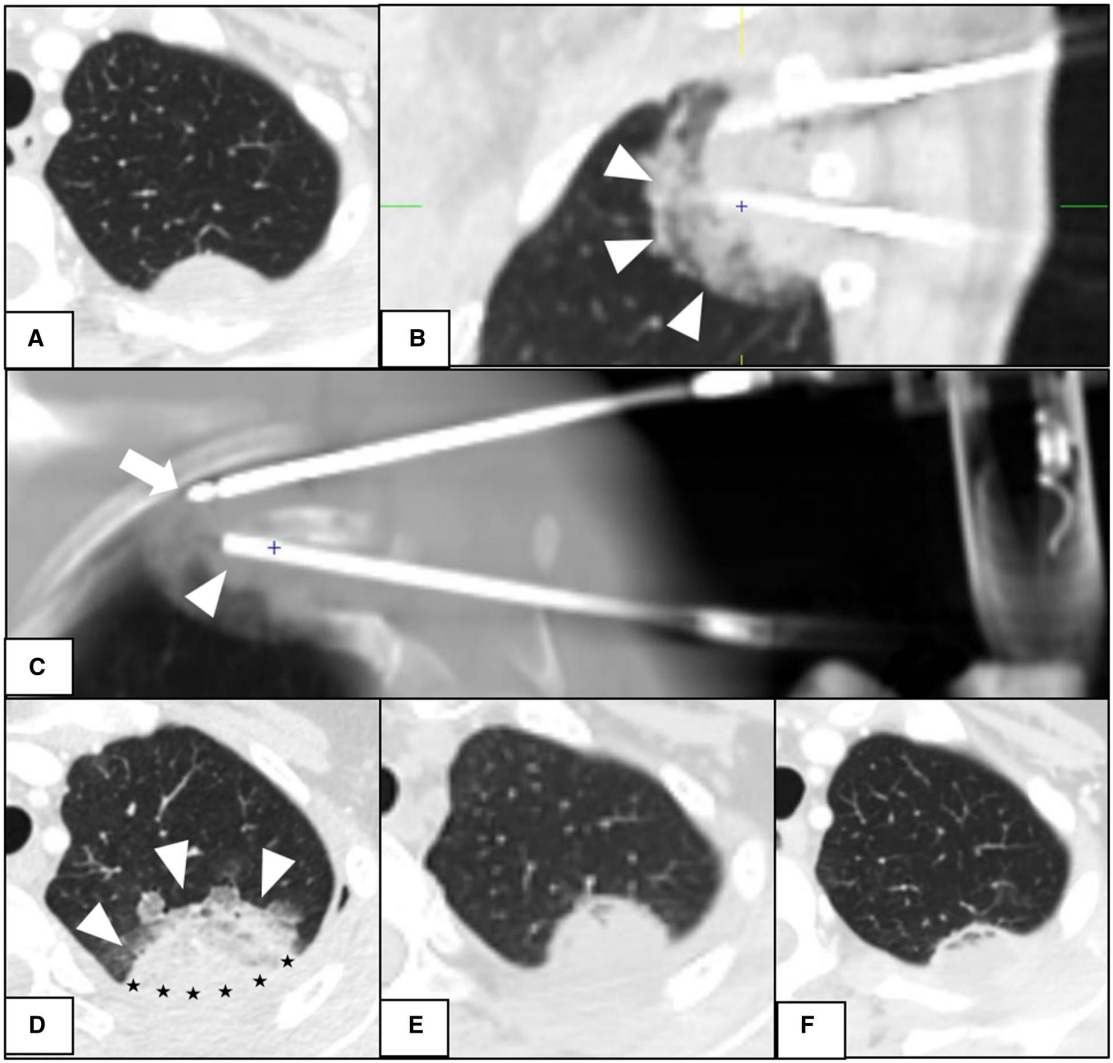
A. lung metastasis, from uterine leiomyosarcoma, with involvement of adjacent pleural layer, relapsed after Radiation Therapy (RT); B. sagittal MPR reconstruction shows the two 10gauge coaxial needle introducers placed into the tumor during cryoablation and the parenchymal effusion surrounding the tumor during treatment (arrowheads); C. Maximum Intensity Projection (MIP) and Multiplanar Reconstruction (MPR) reconstruction shows the cryoprobe (arrow) and cannula (arrowhead) in position; D. parenchymal effusion surrounding the tumor 24 hours later (arrowheads), with reactive pleural effusion (black stars); E. CT scan at 1 month shows initial tumor shrinkage; F. CT scan at 3 months clearly shows further tumor shrinkage. Pleural and parenchymal effusion is no longer visible.
